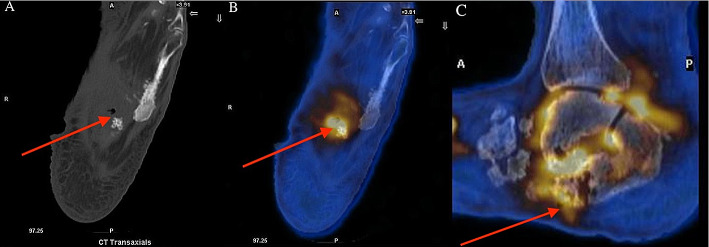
^18^F-FDG-PET/CT of left foot showed intense uptake tracked from a soft tissue ulcer. A-C, PET and non-contrast CT performed 95 min after the administration of ^18^F-FDG revealed intense uptake in the soft tissues, bones, and joints of the left hindfoot/midfoot. FDG uptake tracked from a soft tissue ulcer in the plantar surface of the foot (A-B, arrow) to the adjacent calcaneus (C, arrow)
